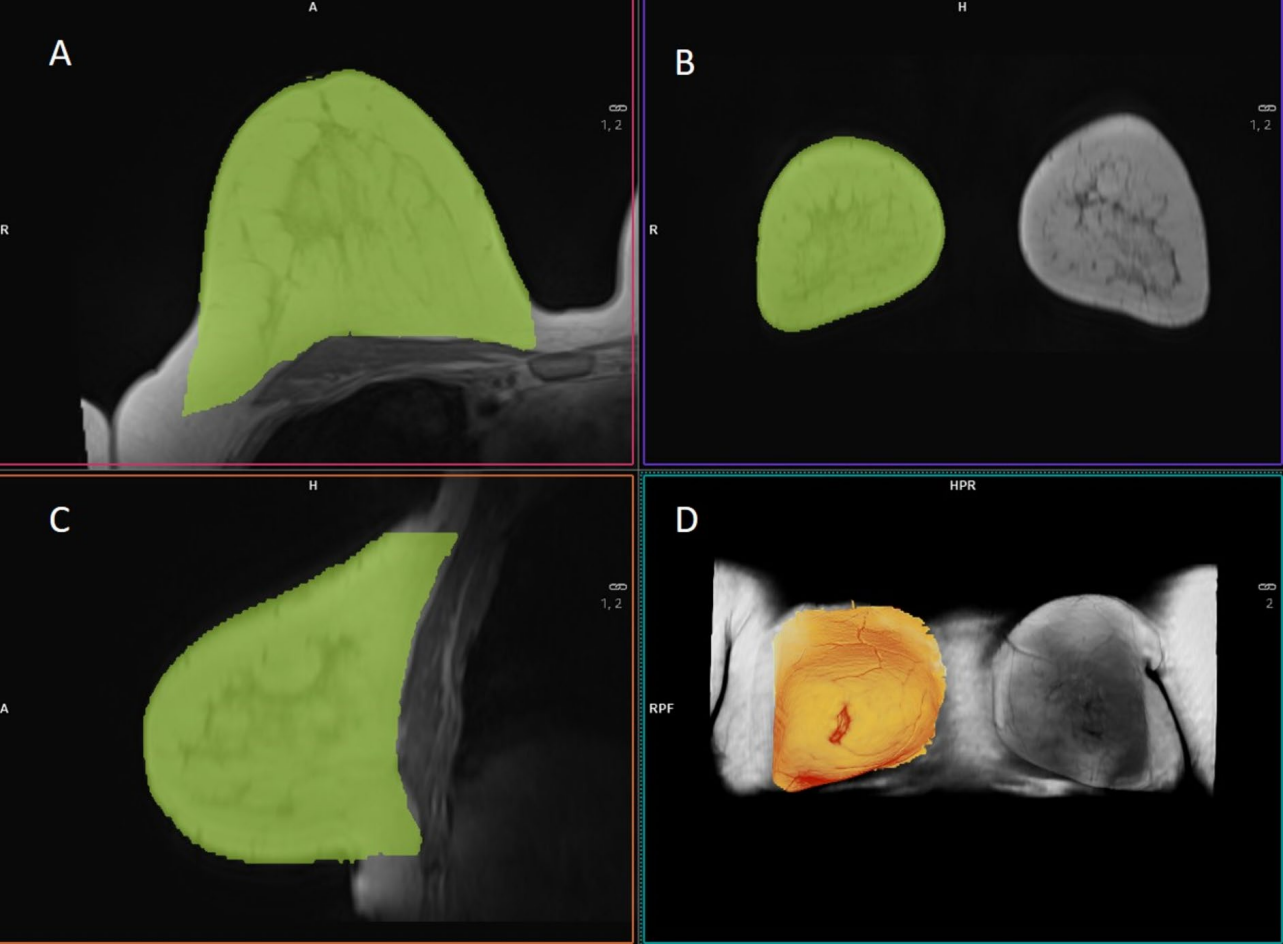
Example of semiautomatic MRI volumetry (MM Reading, Syngo.via, Siemens Healthcare, Erlangen, Germany). A Transversal, B Coronal, C sagittal slice orientation of semiautomatic volumetry of the right breast. D Corresponding VRT dataset in head-feet orientation
